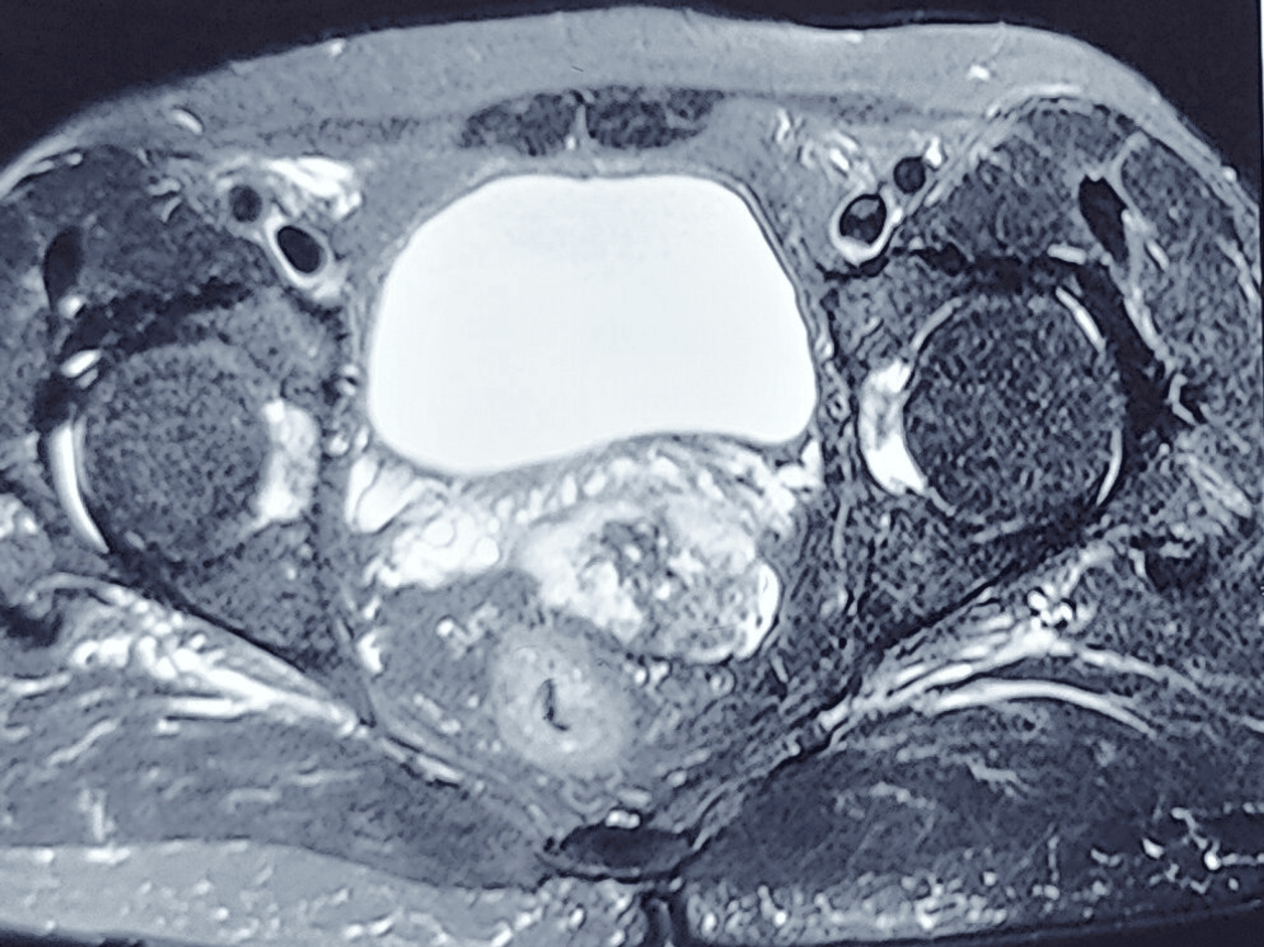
MRI scan on last follow-up showing more than 50% reduction in size and no sign of extra rectal involvement, however; the mesorectum is involved and tumor abuts the seminal vesicle and prostate.
